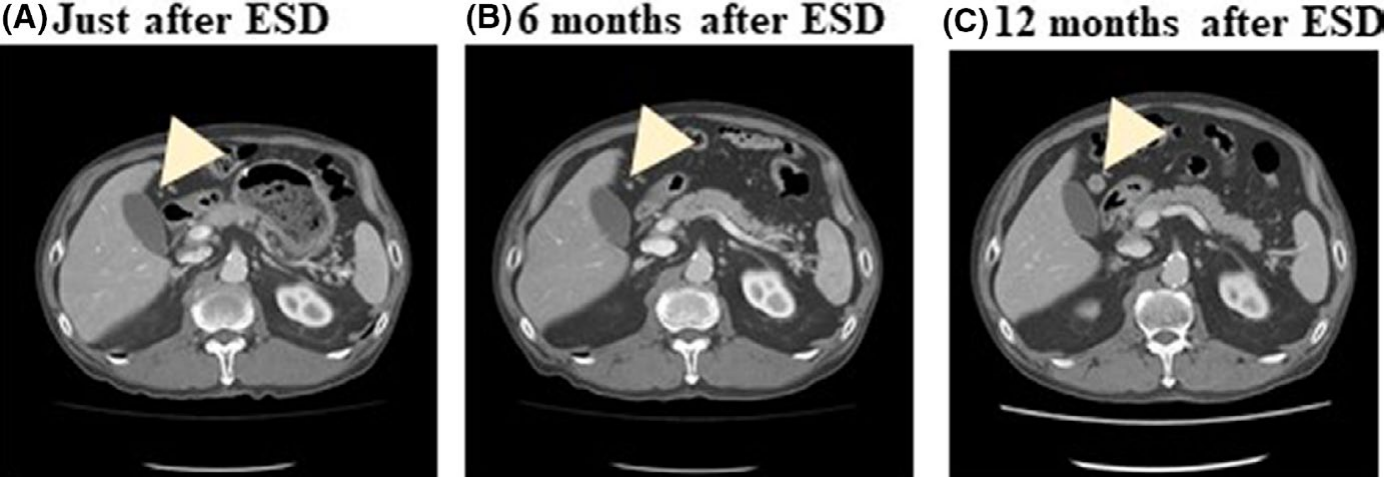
Representative CT images for clinical staging in gastric cancer. Diagnostic difficulties for small lymph node metastases in gastric cancer. (A) Just after ESD, no lymph node swelling. (B) 6 mo after ESD, no lymph node swelling. (C) 12 mo after ESD, 1.2 cm sized lymph node swelling is shown nearby gallbladder. This lymph node is clinically metastatic at the No. 6 station
